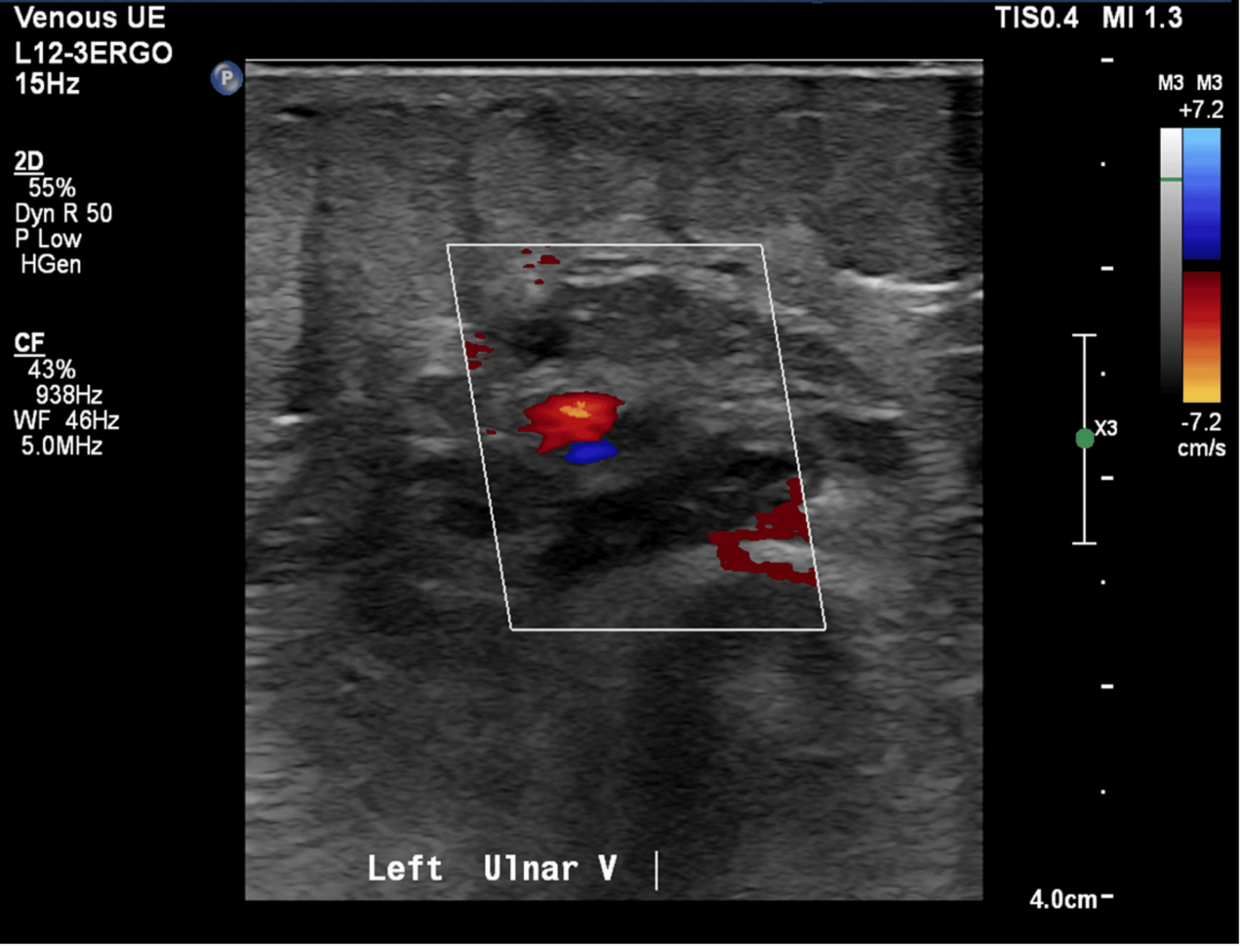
Left ulnar vein duplex ultrasound.
